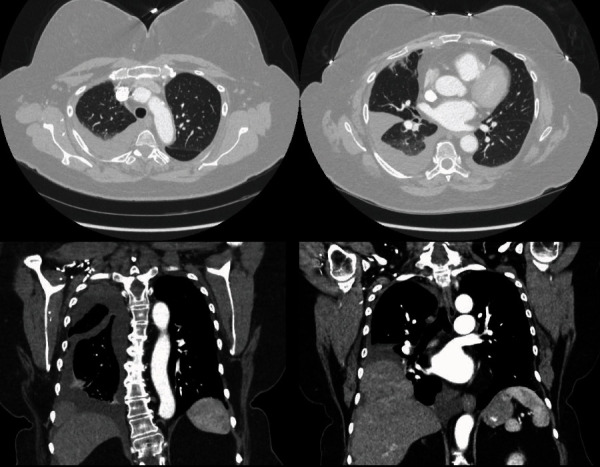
CT chest and abdomen showing right-sided pleural effusion and right lateral diaphragmatic hernia.
